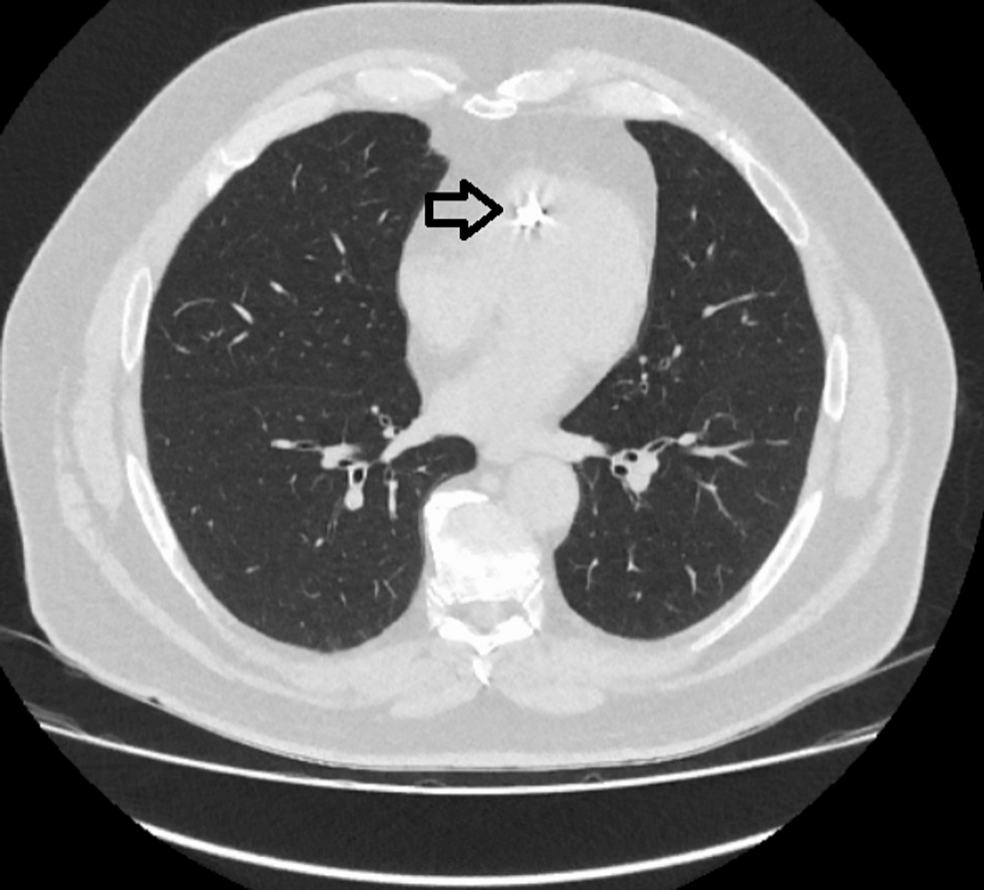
The lung window of the CT chest, with an arrow pointing to the bright dense mass. CT, computed tomography
